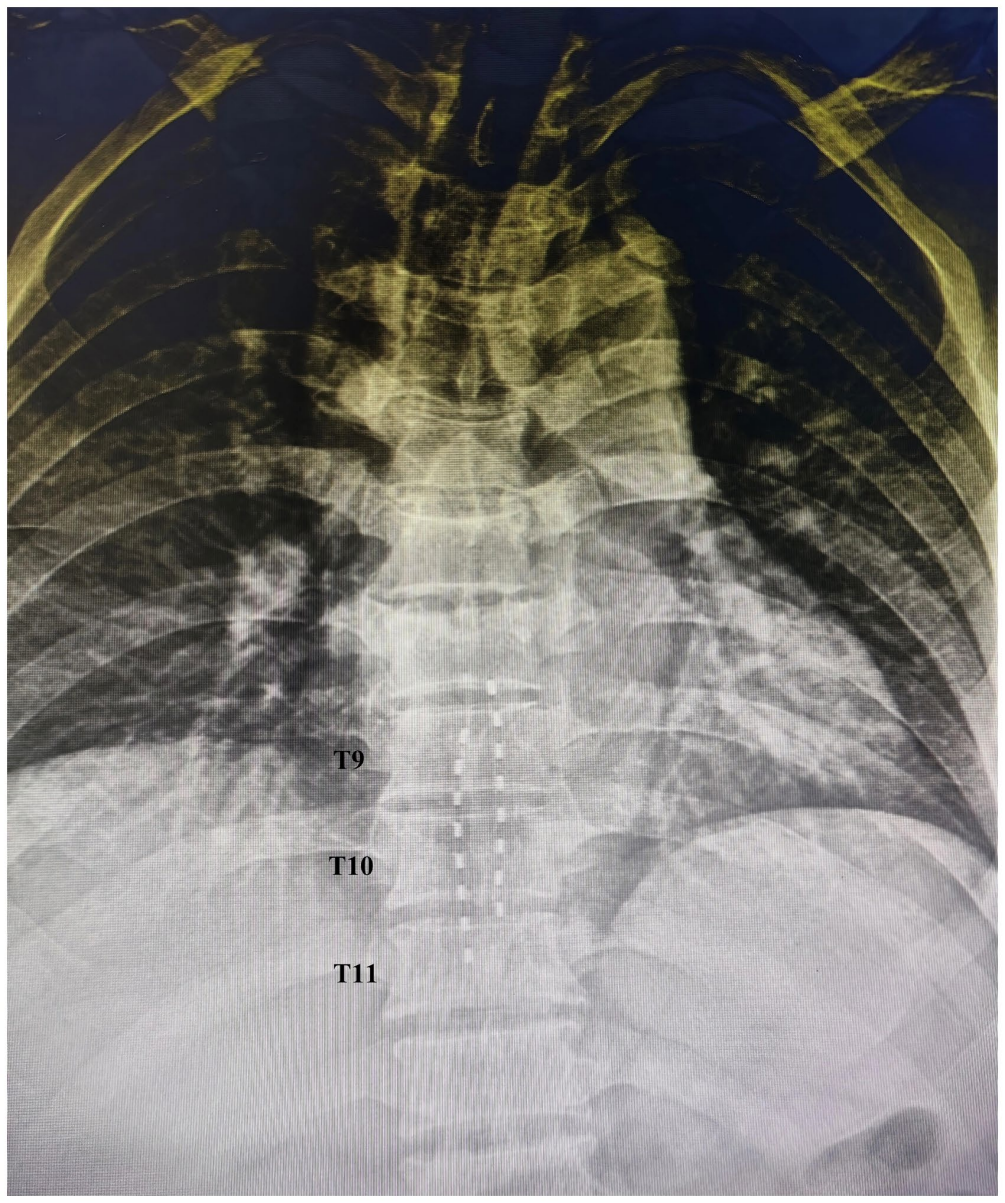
X-ray image of two eight-contact percutaneous leads placed in the T9–T11 level.
